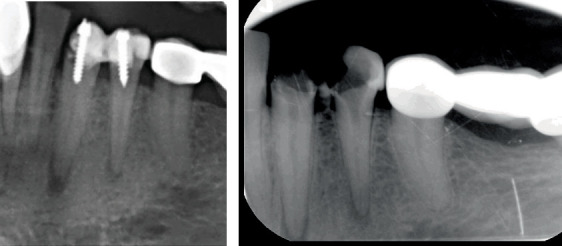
Pretreatment periapical radiograph showing (b) apical radiolucencies in Teeth 33 and 34, along with a (a) metal postcore.
